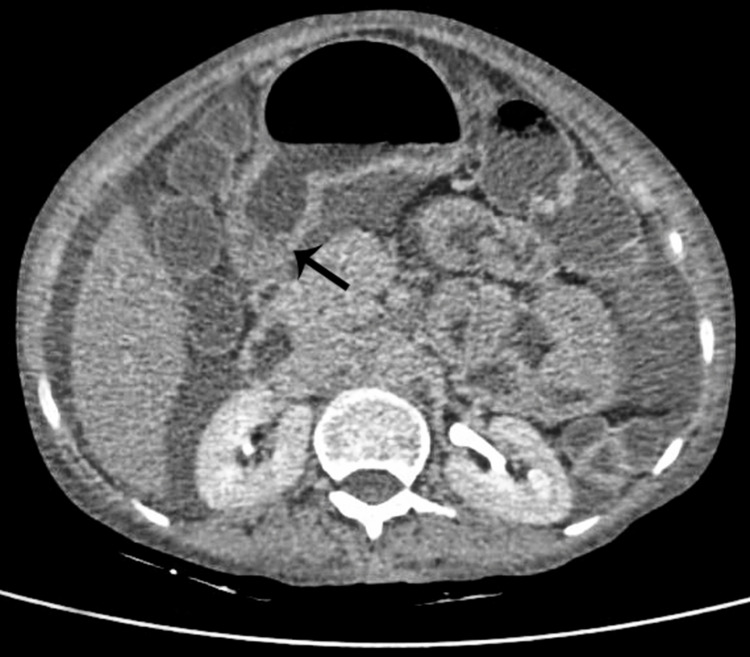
Duodenal wall thickening visible in CT enterography (black arrow)
